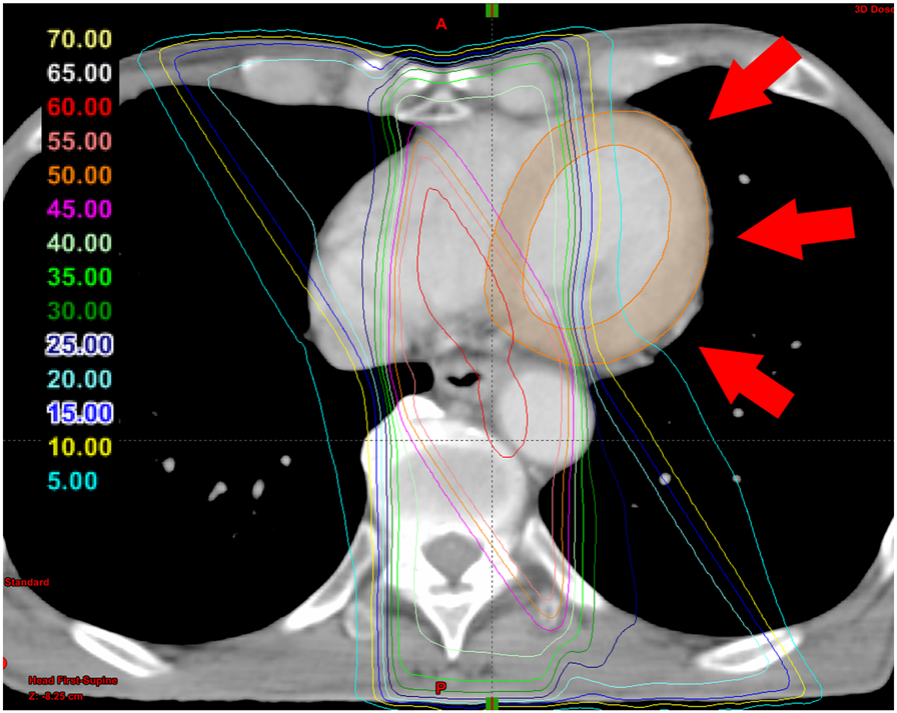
Evaluation of the relations between radiation dose and myocardial metabolic disorder. Normalization was applied on the mean of single photon emission computed tomography counts in regions of the myocardium receiving less than 5 Gy (red arrow). Relative values in each dose region (interval of 5 Gy) were calculated on the basis of this normalization for each patient. The reduction in the percent of relative values was calculated.
